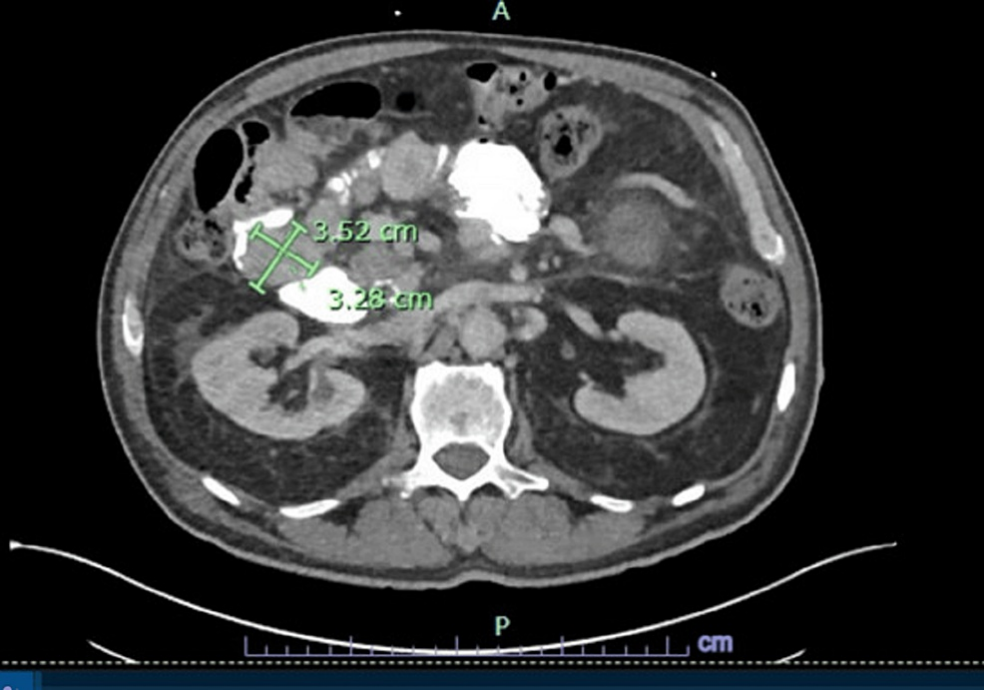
Worsening peritoneal implants on repeat CT abdomen/pelvis with contrast post-small bowel GIST resection in hospital (3.2×3.52 cm).
